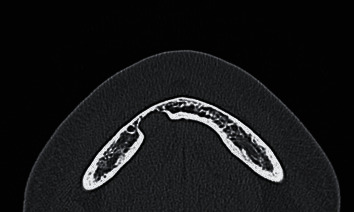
Computed tomography image, horizontal cross section, hard tissue mode. A rounded cavity is observed in the lingual side of the mandible from the right anterior to the premolar region. The margin of the cavity in the lingual side of the mandible is a high-density line, suggesting cortical bone, and is smooth. The mandibular canal is not connected with the cavity.
